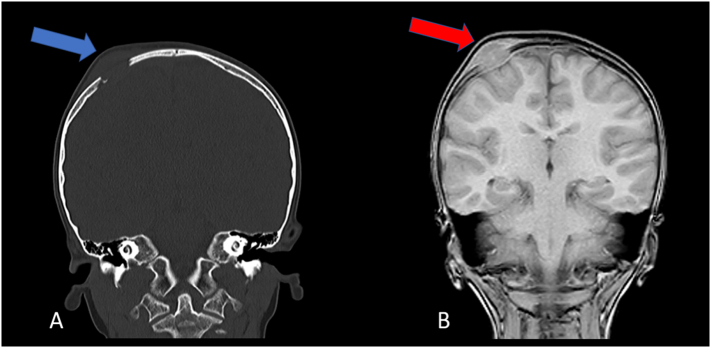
(A) CT scan shows a lytic bony lesion (Blue Arrow). (B) MRI scan shows a 2.5 × 1.5 cm bone lesion extended as swelling (Red Arrow). (For interpretation of the references to colour in this figure legend, the reader is referred to the web version of this article.)
